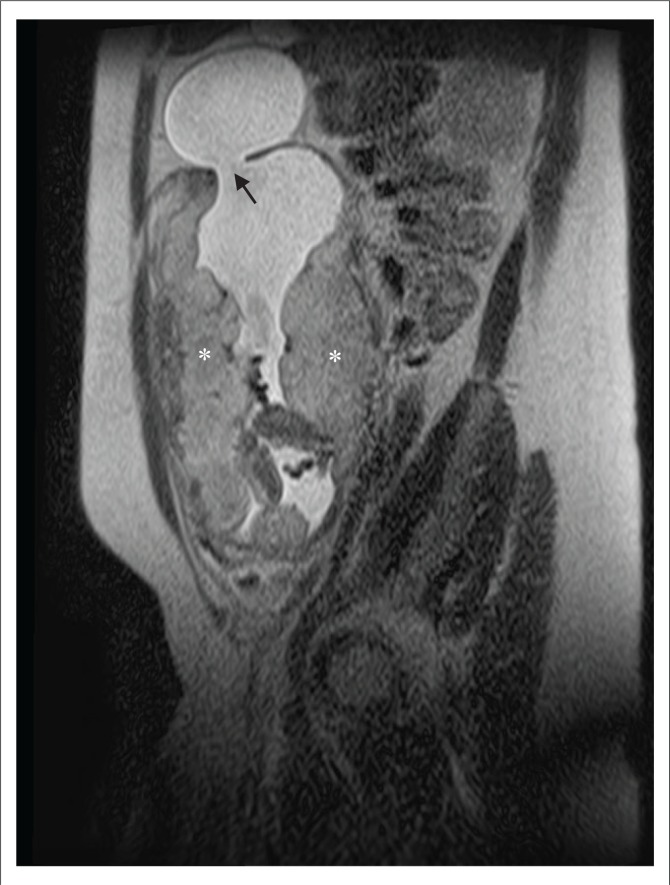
Sagittal T2-weighted magnetic resonance imaging demonstrating a defect in the myometrium of the uterine fundus through which part of the amniotic sac has herniated (arrow). Placental tissue could be seen lining the anterior and posterior uterine walls, and extending to the fundus anteriorly (asterisks).
